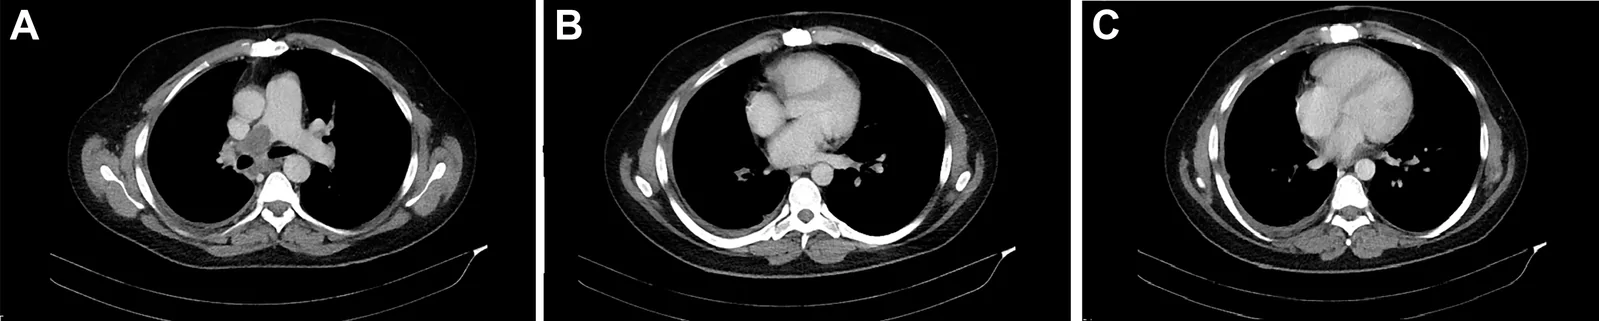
Contrast-Enhanced Computed Tomography Pulmonary Angiography (A) Filling defect within the main pulmonary artery extending into the right pulmonary artery, consistent with acute pulmonary embolism. (B) Complete occlusion of the right pulmonary artery trunk with absence of contrast opacification on the right side, while the left pulmonary artery and its branches demonstrate normal contrast enhancement. (C) Segmental and subsegmental emboli involving the right pulmonary arterial branches, with absence of contrast filling in the right lower lobe branches, whereas preserved contrast opacification is noted in the corresponding left lower lobe branches.
